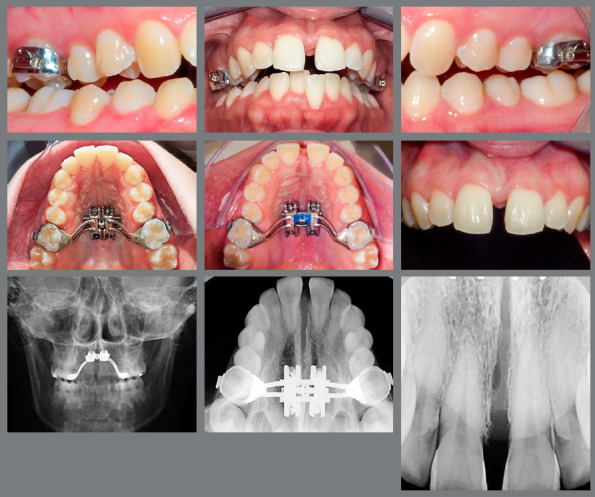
Intraoral and radiograph images after maxillary expansion.
